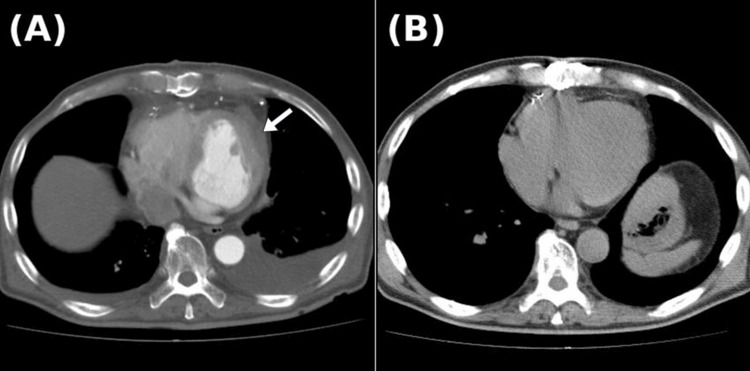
Postoperative computed tomography (CT) images (A) A small amount of fluid collection is seen at the site of foreign body removal during hospitalization (white arrow). (B) Follow-up computed tomography (CT) at six months postoperatively shows complete resolution of the fluid collection.
